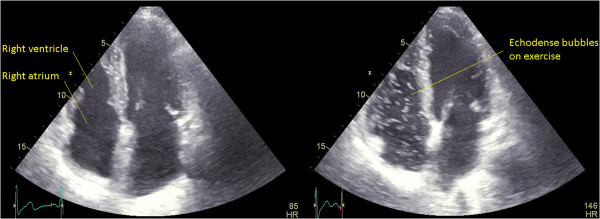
Exertional fat embolism on treadmill stress echocardiography. Apical four-chamber views showing no bubbles in the right heart at rest but an extensive shower of small echodense bubbles in the right heart on treadmill exercise stress testing, consistent with fat emboli. HR, heart rate.
